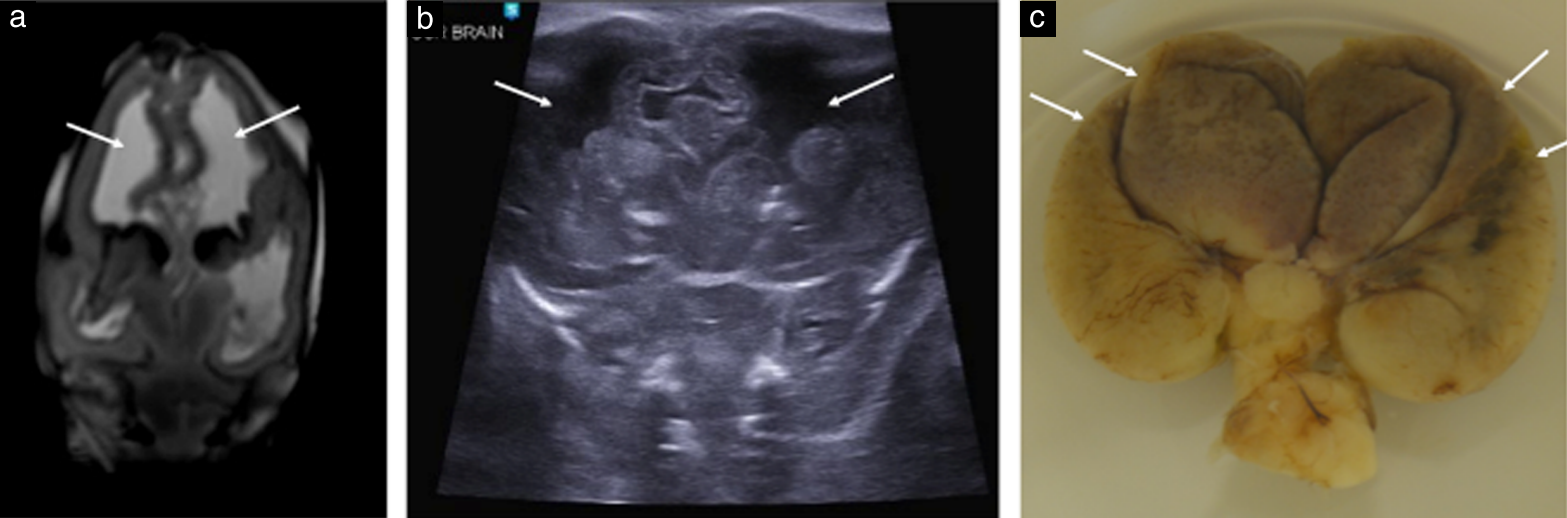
Postmortem imaging in 18‐week fetus with ventriculomegaly, after termination of pregnancy: example of concordant diagnosis between postmortem ultrasound (PM‐US) and postmortem magnetic resonance imaging (PM‐MRI), with autopsy confirmation. (a) Coronal T2‐weighted PM‐MRI image through foramen of Monroe, demonstrating bilateral ventriculomegaly (arrows). (b) Corresponding coronal image of brain on PM‐US demonstrating ventriculomegaly (arrows). (c) Macroscopic photograph of extracted brain, taken in water bath at autopsy, demonstrating dilated, ‘baggy’‐appearing cerebral hemispheres (arrows) in keeping with underlying ventricular dilatation.
